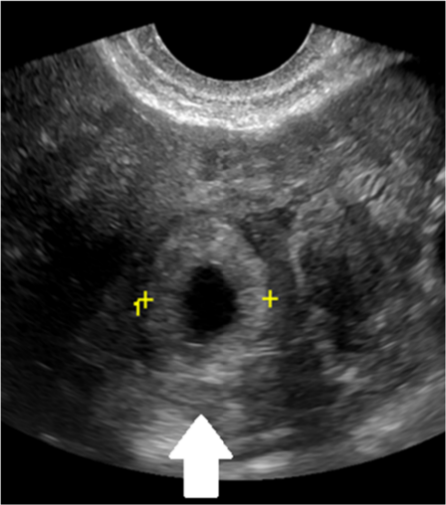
Tubal ectopic pregnancy by transvaginal ultrasound. The arrow indicates the ectopic gestation with a surrounding hyperechoic ring, called the ‘bagel’ or ‘tubal’ sign
